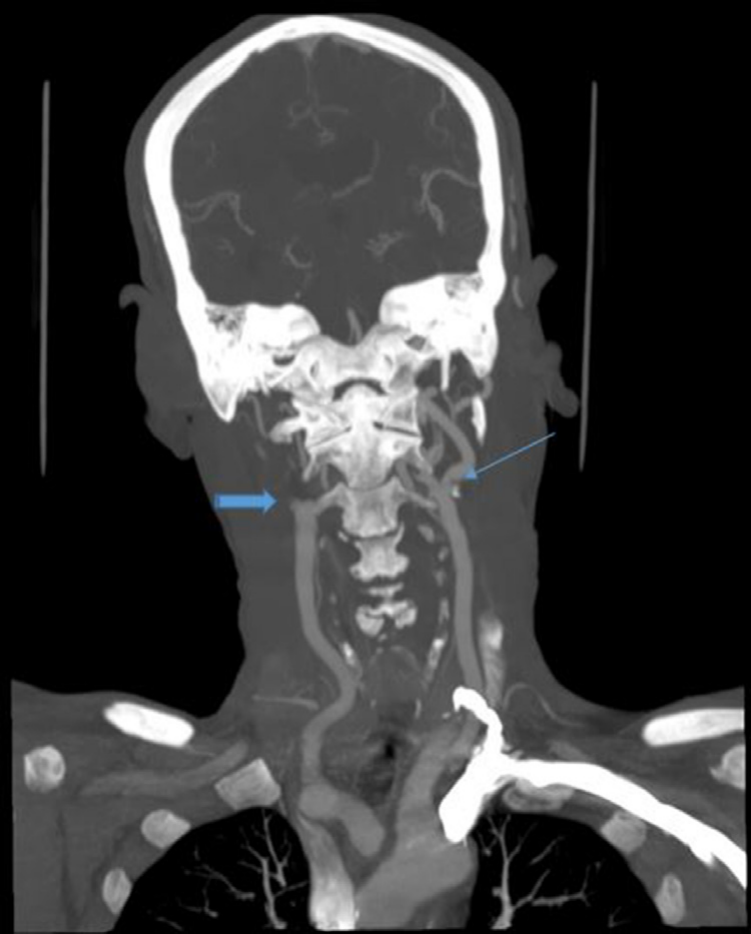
Supra-aortic arteries CT scan showed an occlusion of the right internal carotid artery (striped right arrow) and left bulbar.
